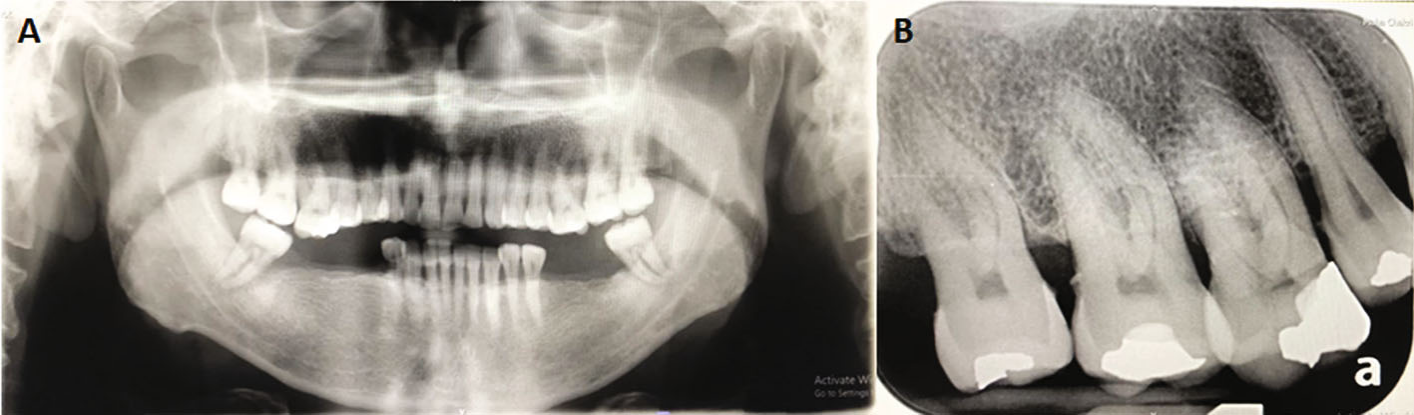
Panoramic radiograph shows no sign of intrabony tumor (A). Periapical radiograph shows generalized vertical bone resorption suspected due to chronic periodontitis and furcation involvement at right maxillary molar teeth (B).
